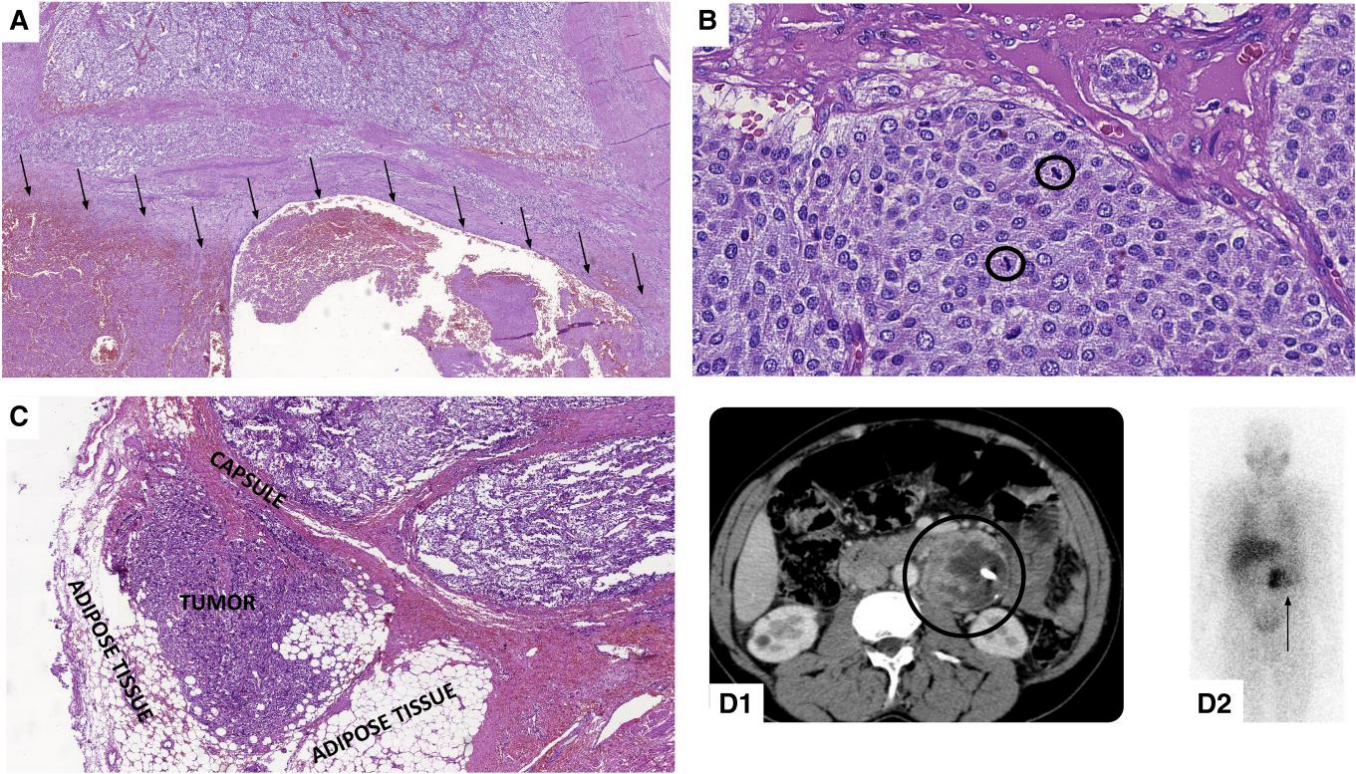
Predictors of metastatic disease of prognostic score in paragangliomas (PSPGL). A, Tumor with extensive area of necrosis (underside—arrows). B, Tumor with high mitotic index (2 mitosis/1 HPF). Circle: mitosis. C, Tumor with extension into adipose tissue. D, Extra-adrenal paraaortic paraganglioma (left) seen on computed tomography (circle) (D1) and in metaiodobenzylguanidine scintigraphy (MIBG) (arrow) (D2).
